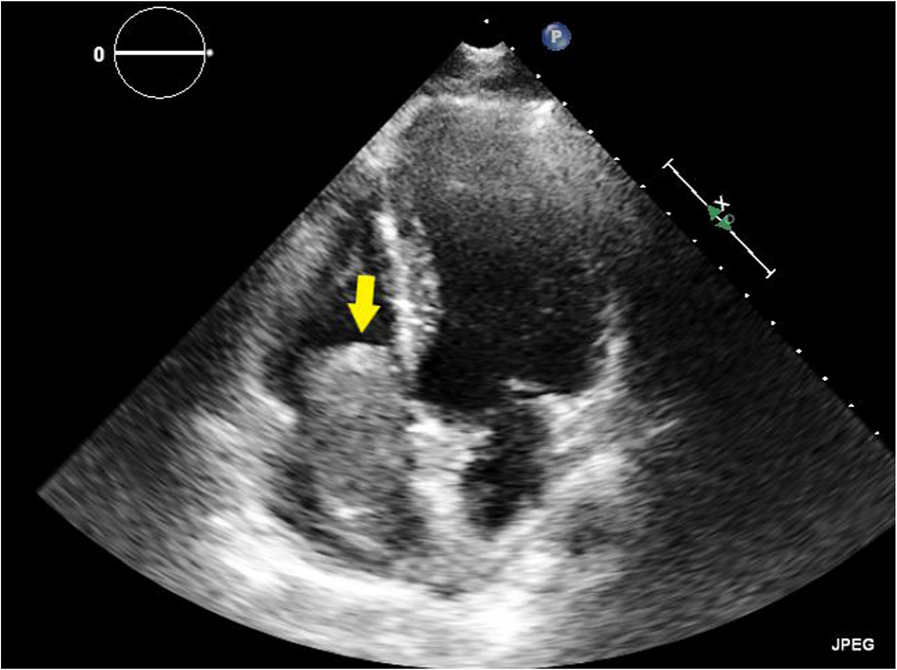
Transthoracic two-dimensional echocardiography, apical 4 chamber view showing a huge right atrial mass (about 8 × 7 cm), filling the whole cavity of the right atrium
